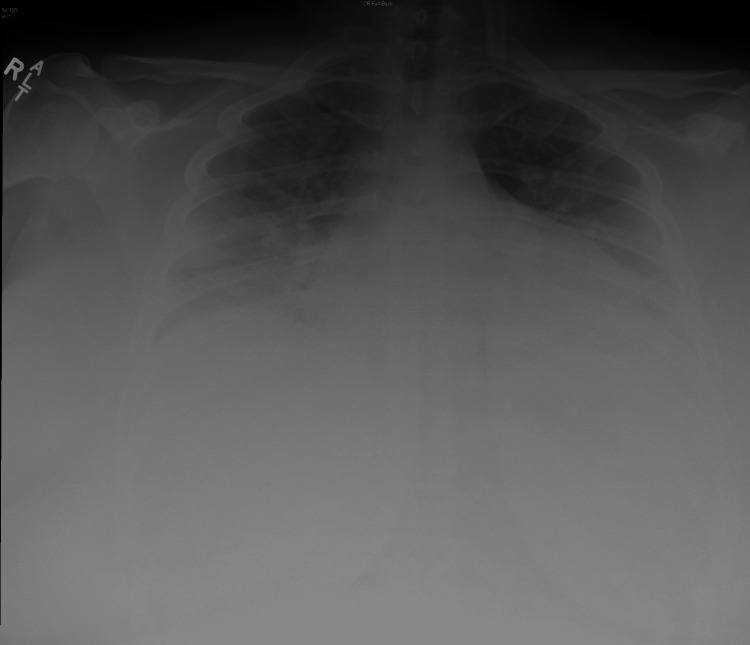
Chest radiograph on the day of ER visit demonstrating bilateral infiltrates and pneumonia
